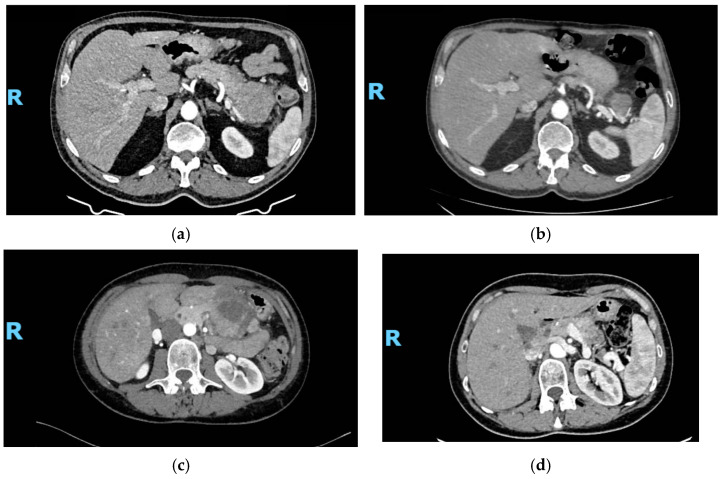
Best response in two patients treated with FOLFOX + SSA: (a–c) CT-scans at baseline; (b–d) CT-scans after VI cycles. R: right.
